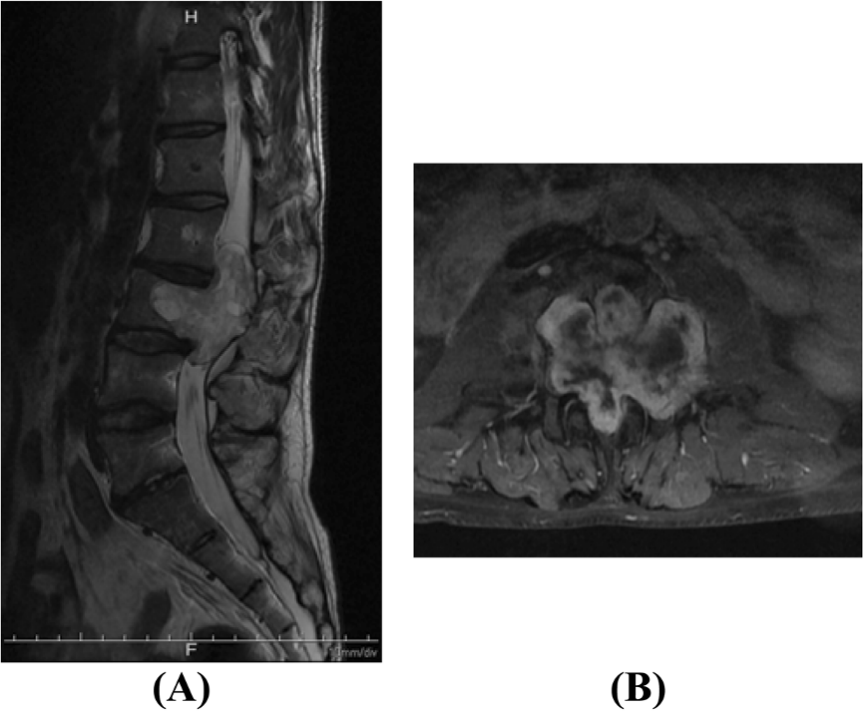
Pre-operative magnetic resonance imaging scans of the lumbar spine. A large tumor mass in the spinal canal at L2-L3 and the vertebral body at L3 can be visualized with increased signal intensity on a sagittal T2-weighted image (A) and a gadolinium-enhanced T1-weighted image (B).
